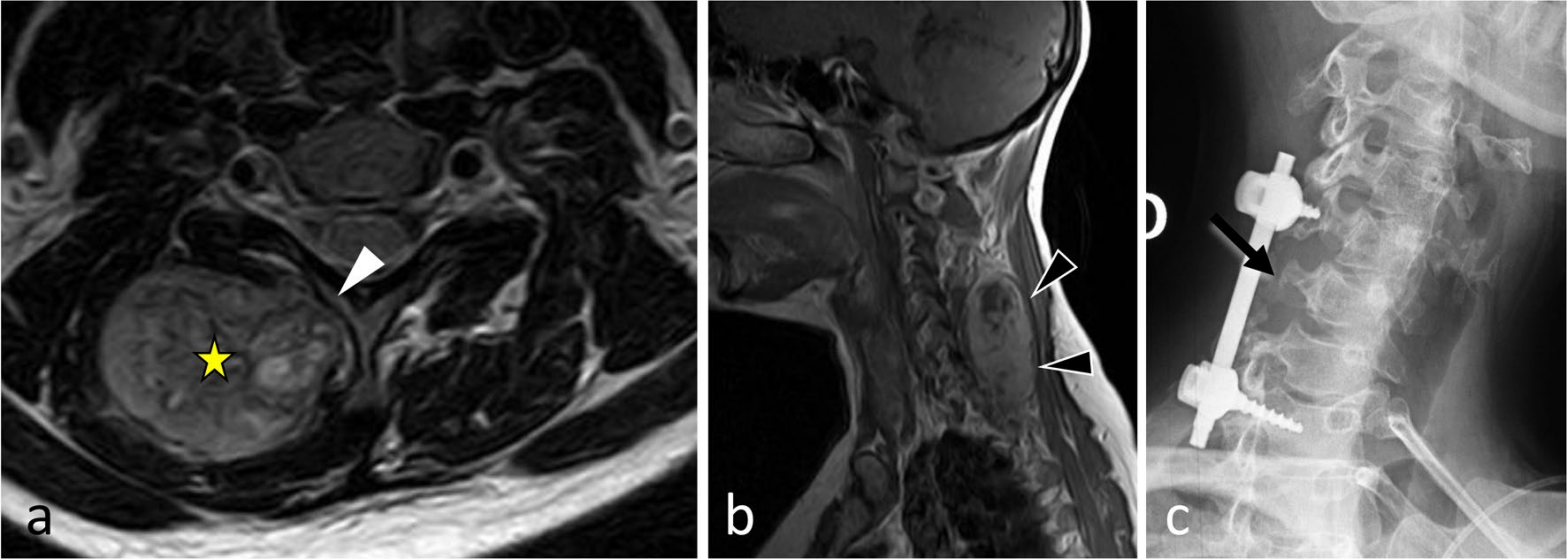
Mesenchymal chondrosarcoma* of right hypaxial muscles in a 37-year-old female with chronic cervical pain and bump in the neck. MRI shows a lobulated mass with high signal on axial T2WI (a) and heterogeneous enhancement pattern after contrast injection (black arrowheads, b). White arrowhead demonstrates the disappearance of the fat plane separating the mass and the neural arch. The yellow star shows the epicentre of the mass. Cervical radiograph (oblique view) shows posterior cervical fixation following laminectomy (c)
